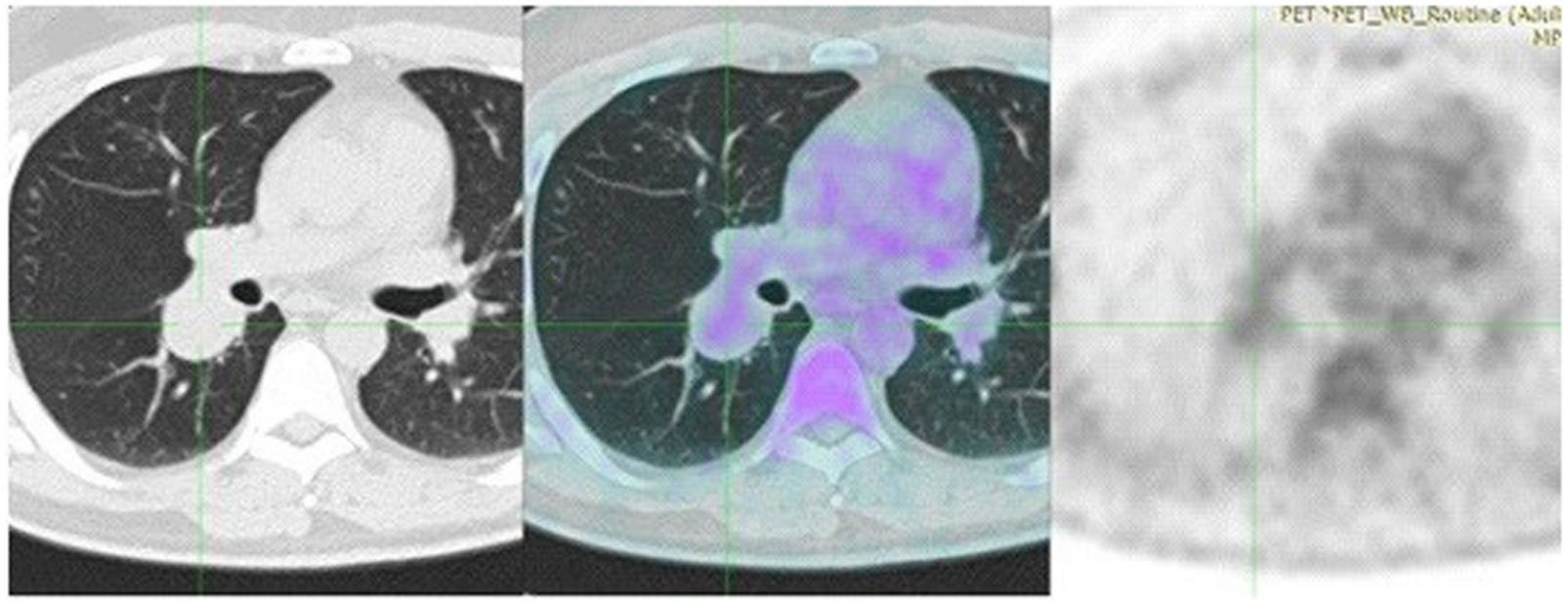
Positron-emission tomography (PET) demonstrates a diffuse, low FDG uptake (SUV_max_ 2.1) of the lesion, which exceeded that of vascular structure of the mediastinum (SUV_max_ 1.7).
